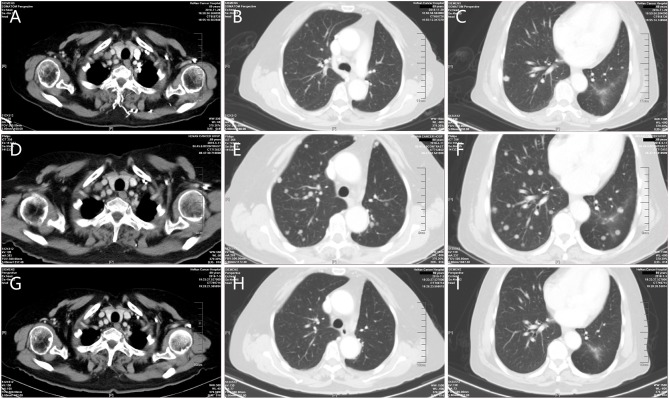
Representative computed tomography (CT) obtained throughout the clinical course. (A–C) CT during initial therapy (prior to the first time of pembrolizumab administration). (D–F) Progressive disease while receiving pembrolizumab therapy. (G–I) Significant reduction in tumor burden following the fourth dose of pembrolizumab after re-administration.
